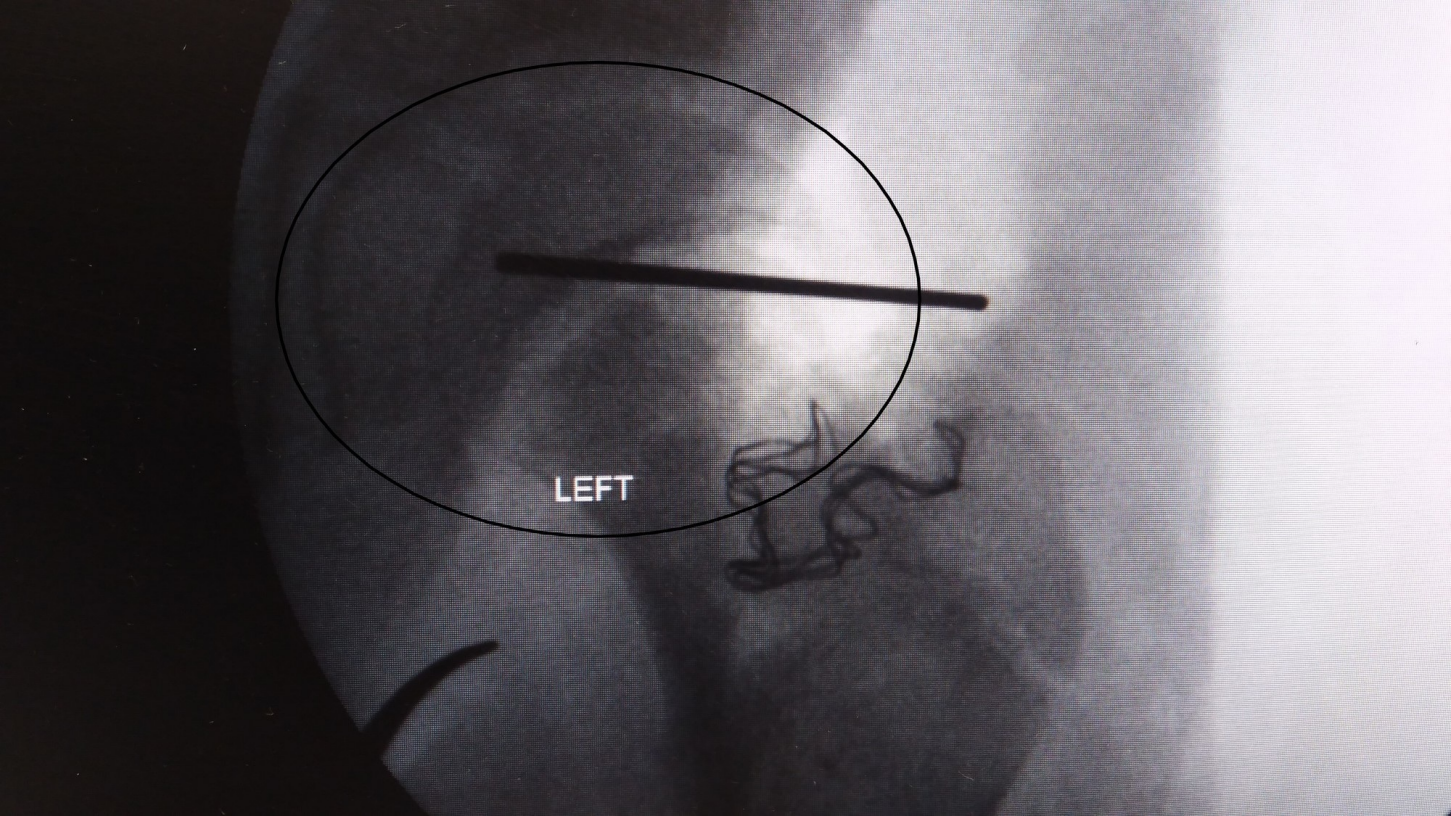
X-ray left hip intra-operative. X-ray left hip showing reduced with Kirschner wire.
